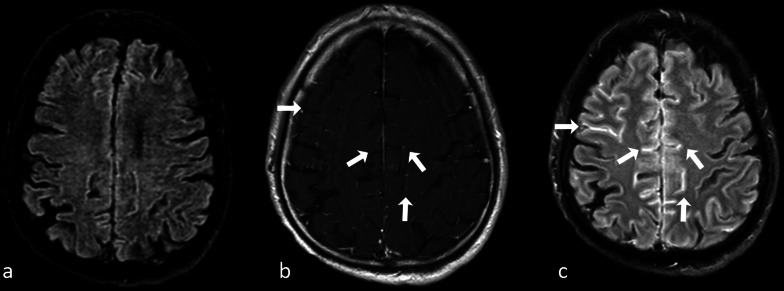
25-year-old female patient with suspected meningitis. Nonenhanced fluid attenuated inversion recovery (FLAIR) a image appears negative. Turbo spin echo (TSE) T1-w. magnetization transfer scan b is inconclusive, although mild effacement of some frontal and parietal sulci can be suspected (arrows). On contrast-enhanced FLAIR (c) scan extensive hyperintensity of several sulci can be easily diagnosed (arrows)
